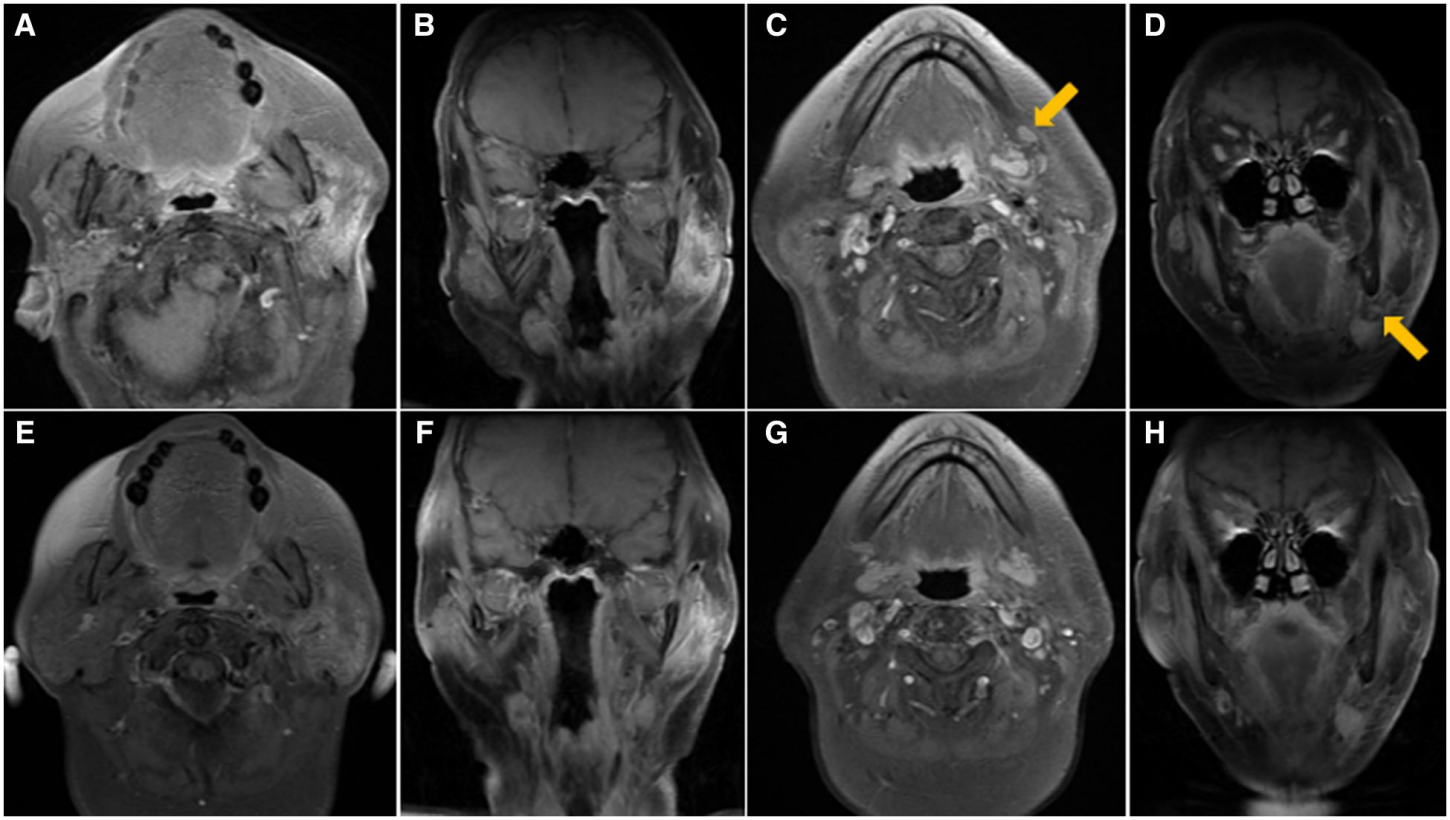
Post-contrast T1 weighted MRI with fat suppression performed 3 months (A-D) and 5 months (E-H) after completion of radiotherapy. First MRI shows significant decrease in size of the left parotid tumor ((A) axial, (B) coronal) and left cervical nodal metastases (arrows, (C) axial, (D) coronal). Second MRI shows further decrease in size of the left parotid tumour ((E) axial, (F) coronal) and resolution of the left cervical nodal metastases ((G) axial, (H) coronal).
